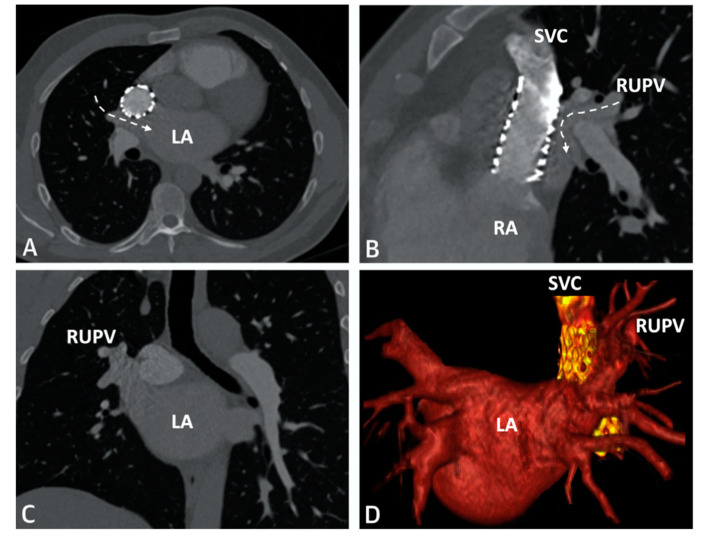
CT imaging following successful covered stent placement demonstrates no pulmonary venous obstruction to the LA (A–C). A 3D virtual model confirms unobstructed drainage from the pulmonary veins to the LA (D). Revised from ref [4].
